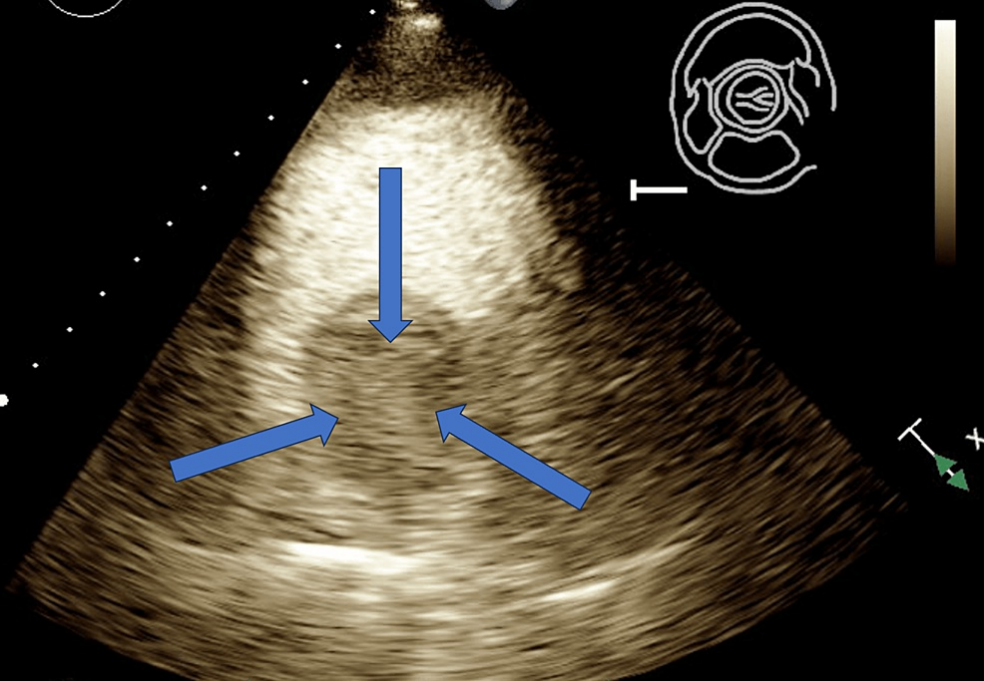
TTE parasternal short axis view at the level of the aortic valve with IV contrast depicting partially vascularized right atrial mass concerning myxoma (blue arrows) TTE: transthoracic echocardiogram
